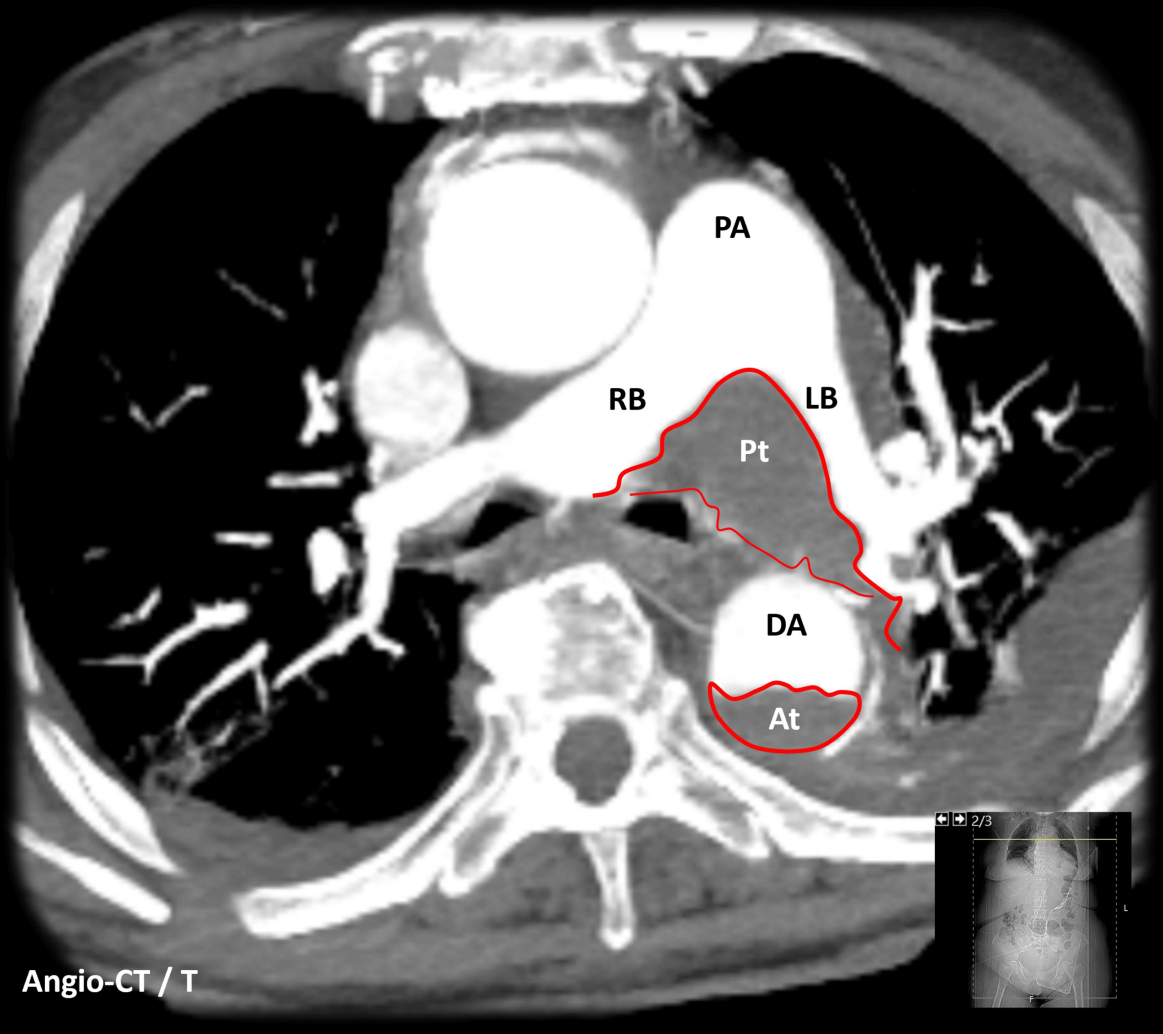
Chest angio computed tomography (transversal reconstruction) At - Aortic Thrombosis; DA - Descending Aorta; LB - Left Branch; PA - Pulmonary Artery; Pt - Pulmonary Thrombosis; RB - Right Branch
